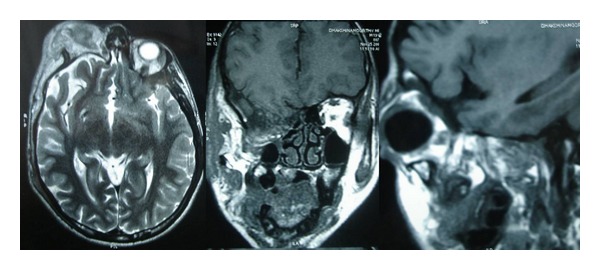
MRI of the brain axial, coronal, and sagittal views showing the absence of right sphenoid wing with herniation of the temporal lobe.
